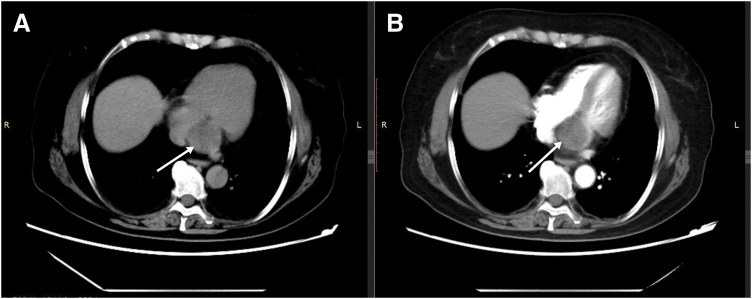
Contrast-enhanced CT scans. (A) Non-contrast image showing a round, heterogeneous hypodense lesion approximately 4.2 cm in diameter within the interatrial septum, with an arrow pointing to the mass. (B) Contrast-enhanced image demonstrating mild enhancement of the mass with partial protrusion into the left atrium; the arrow indicates the tumor.
